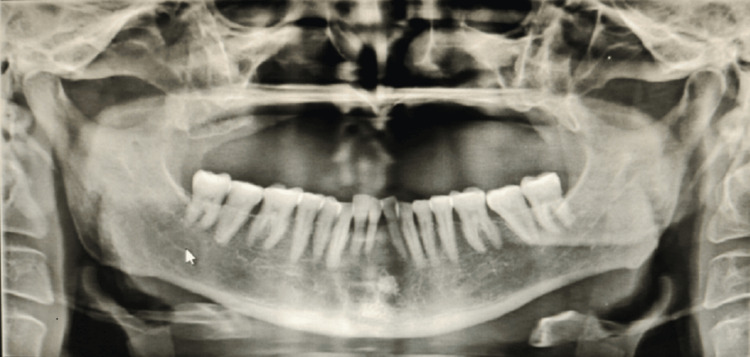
The panoramic radiograph showing the entire radiolucency in the maxillary region.
